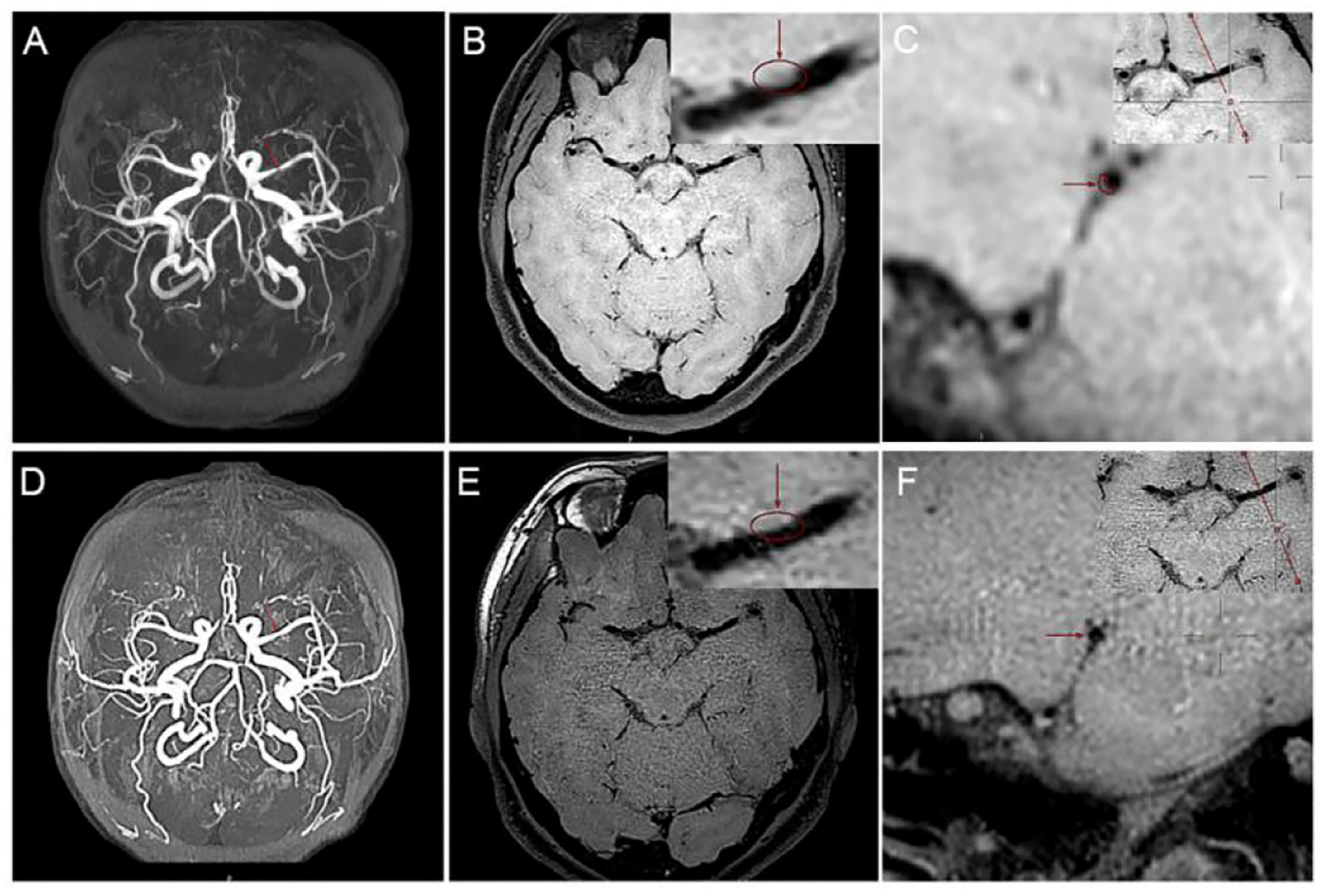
High-resolution MRI (HR-MRI) examination at hospitalization. (A) Time of flight (TOF) magnetic resonance angiography (MRA) showed severe M1 stenosis in the left middle cerebral artery (MCA). (B) T1-weighted volume isotropic turbo spin-echo acquisition (T1W-VISTA) showed the location of the plaque and thickens the eccentric canal wall. T1W-VISTA also showed no significant enhancement on the plaque or surface, similar to the surrounding tissue signals, indicating no thrombosis on the surface of the plaque. (C) 3D-VISTA reconstruction was carried out at the maximal-lumen-narrowing site, and the rough shape of the patch was hand-drawn. HR-MRI examination after 24 months. (D) TOF MRA showed that the degree of lumen stenosis was significantly reduced. (E) T1W-VISTA showed that there were still some plaques on the tube wall, and the lumen was slightly narrow. (F) 3D-VISTA reconstruction showed the diameter of the tube was significantly larger.
